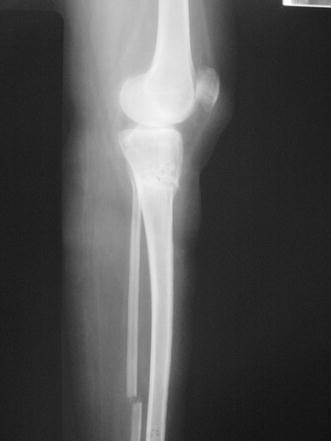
Post-operative plain X-ray showing correction of procurvatum deformity of the knee (lateral view)
